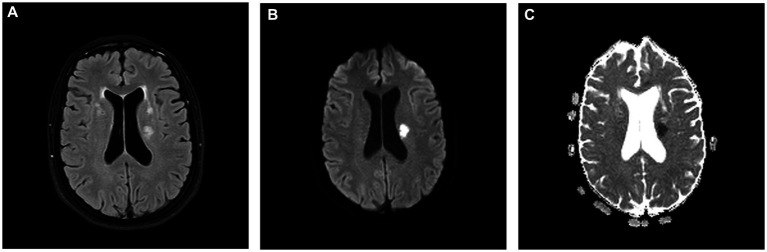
Brain MRI. Acute ischemia of the left corona radiata. (A) FLAIR transverse image, (B) DWI transverse image, (C) ADC transverse image.
